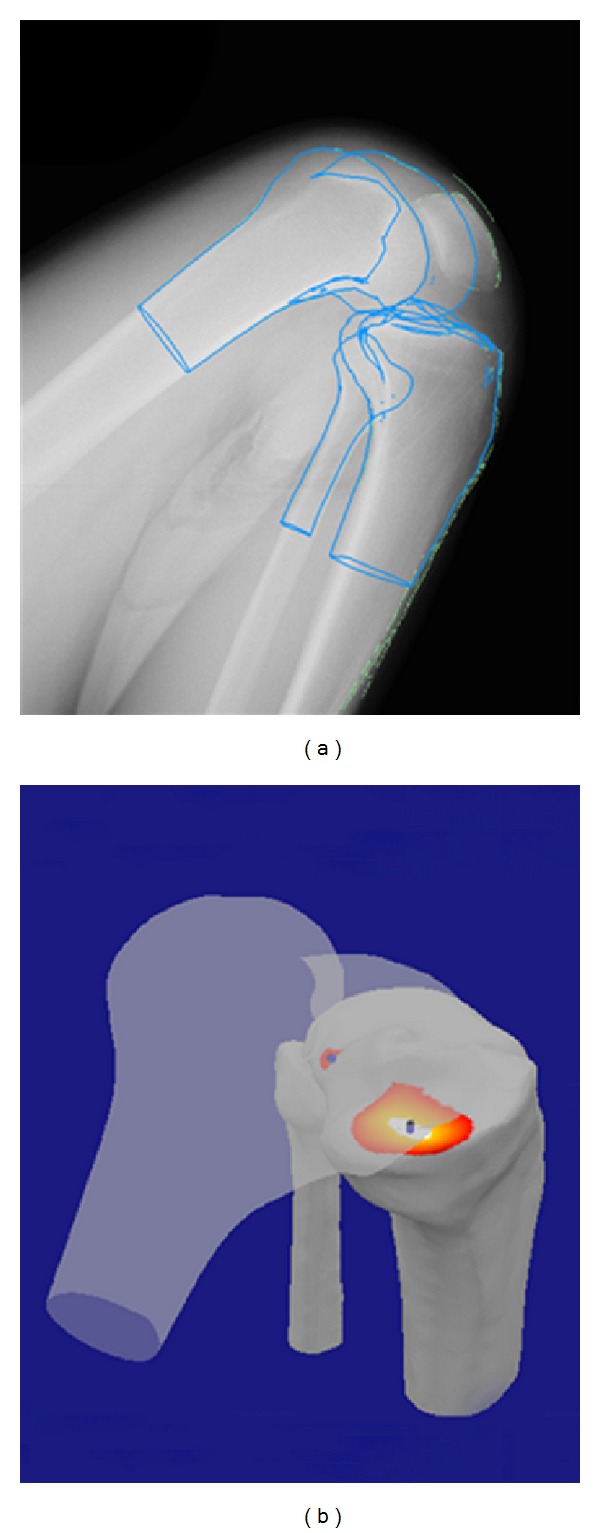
3D-to-2D CT model-to-flat panel image registration techniques were used to determine the *in vivo* healthy knee kinematics (a). Medial and lateral tibiofemoral contacts were computed as the geometric center of the region having less than 6 mm tibiofemoral separation (b).
